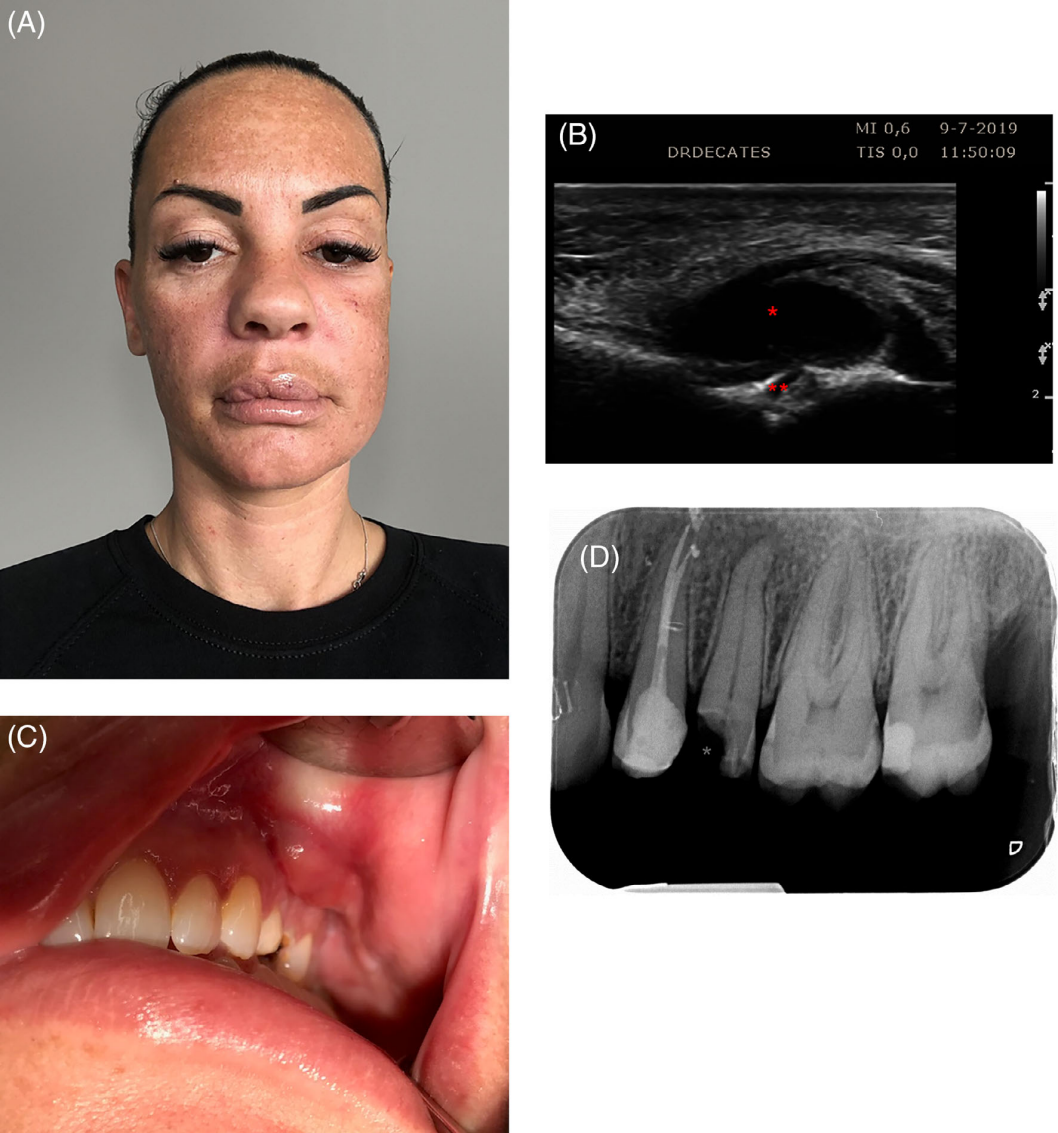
A, Appearance of the edema of the left cheek at the initial visit. B, Ultrasound image of the left cheek clearly showing a hypo‐echogenic area, suspect for abscess and possibly a fistula. C, An abscess and decayed second bicuspid in the left side of the upper jaw. D, Radiological examination of tooth nr 25 showing deep caries with a decayed part of the crown
